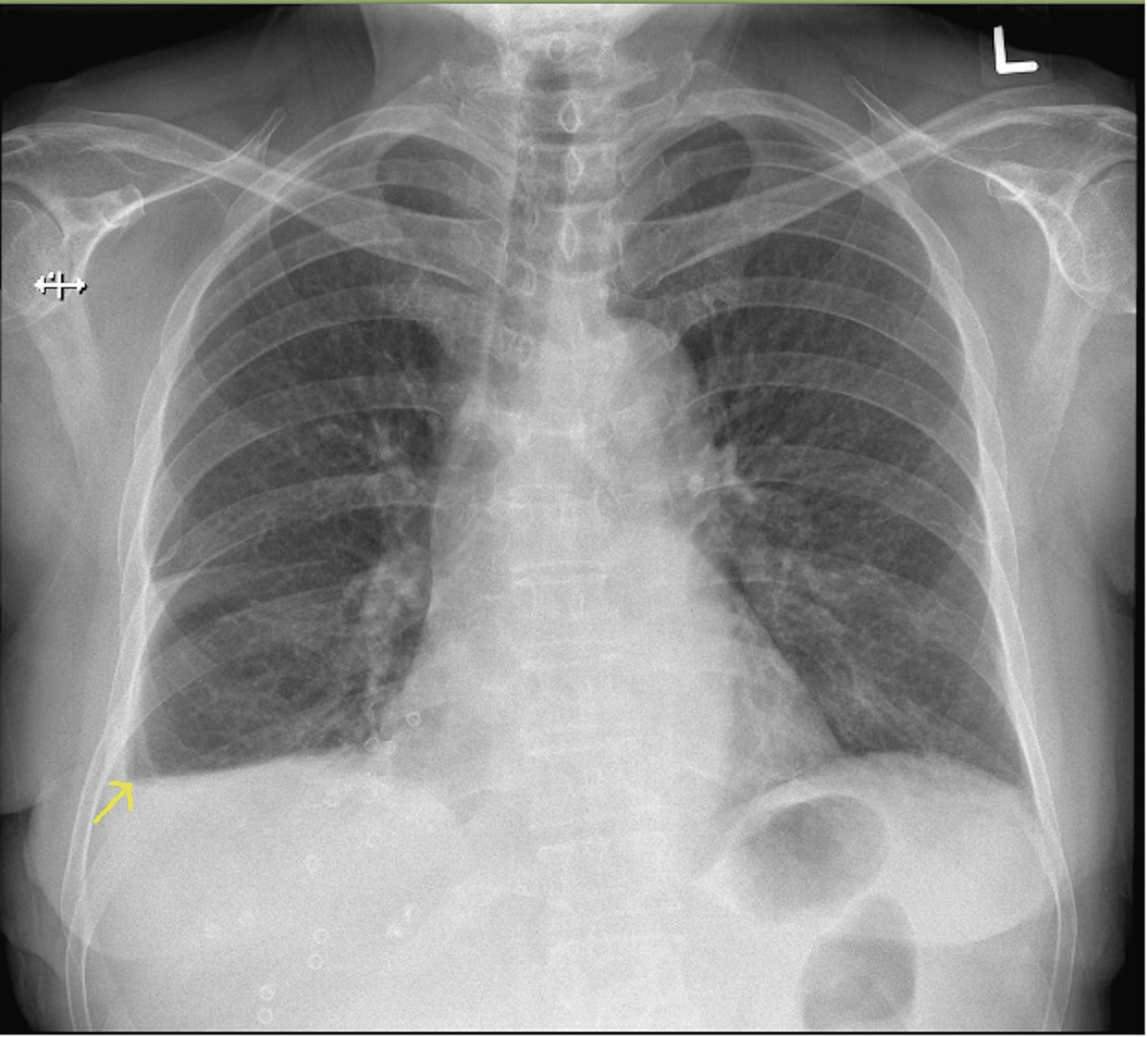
Chest X-ray one month postoperatively demonstrating minimal right pleural effusion.
